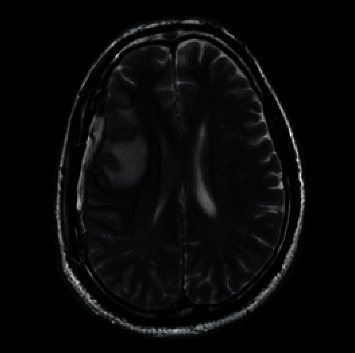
Axial T2-weighted MRI brain showing a subdural collection with mixed density along the right convexity.
